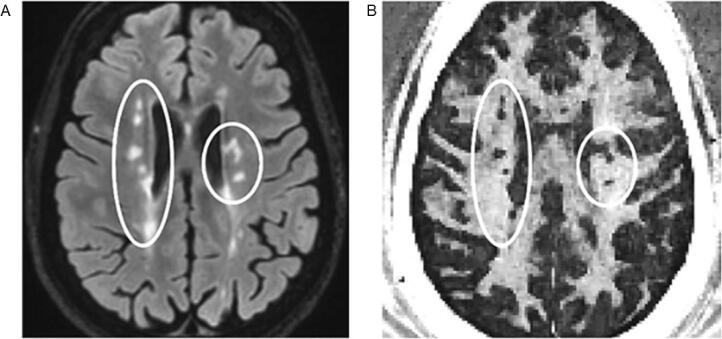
A) T2-weighted FLAIR and B) ultrashort TE subtraction images in a 45-year-old woman with MS, indicating a lower signal intensity in the UTE image in lesions that appear hyperintense on FLAIR. Adapted with permission from Ma et al., 2020.
